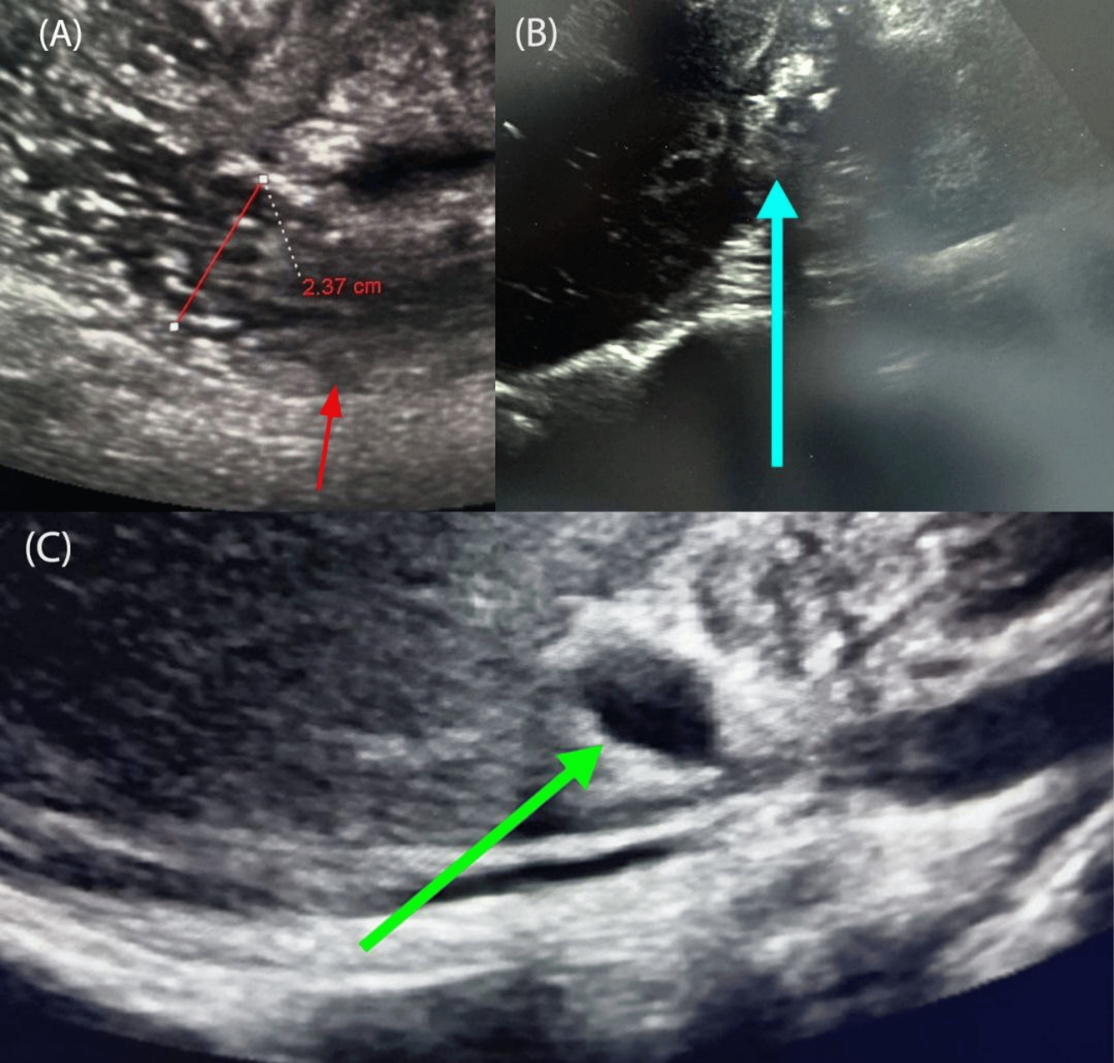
Abdominal ultrasound (A) showing dilated bowel loop (red arrow) and (B) contracted gallbladder with calculi (blue arrow), and (C) echogenic foci with "dirty" shadowing in the biliary tree (pneumobilia) identified by green arrow
